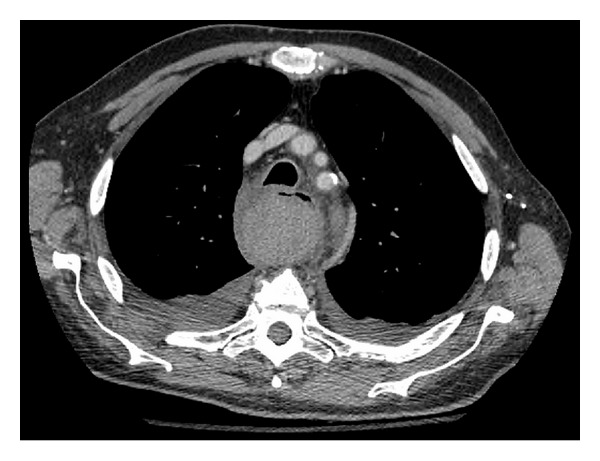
Repeat CT scan of a Posterior Mediastinal Hematoma measuring 4.6 cm × 4.6 cm × 18.5 cm, two days after patient presentation to MGH.
